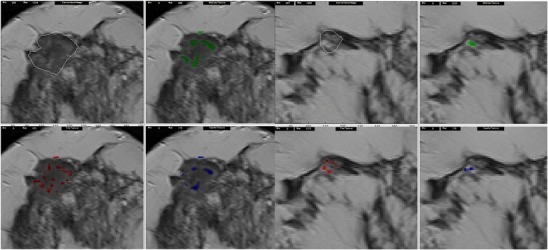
Changes in breast tumor texture following neoadjuvant chemotherapy on T2W-weighted MRI. Tumor shrinkage and an increase in homogeneity are noted following completion of chemotherapy (*right*)
